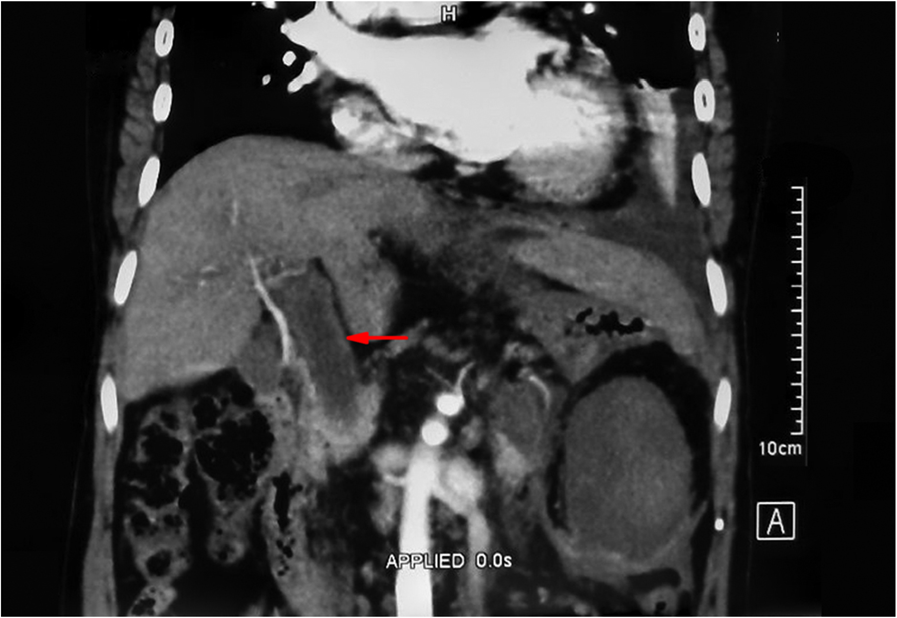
CT scan showed that patients was complicated with portal thrombosis at 4.5 months post-operatively
